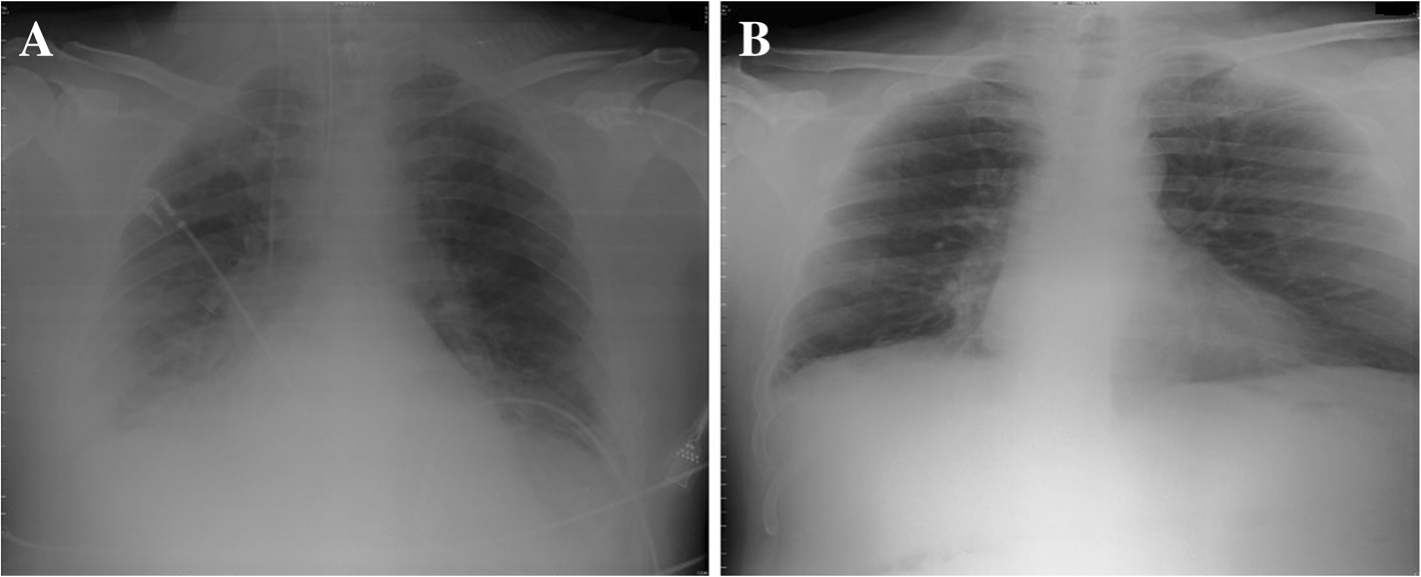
a Anteroposterior chest x-ray obtained in the intensive care unit showing basal pulmonary infiltrate. b Posteroanterior chest x-ray taken prior to discharge and showing disappearance of the pulmonary infiltrate
